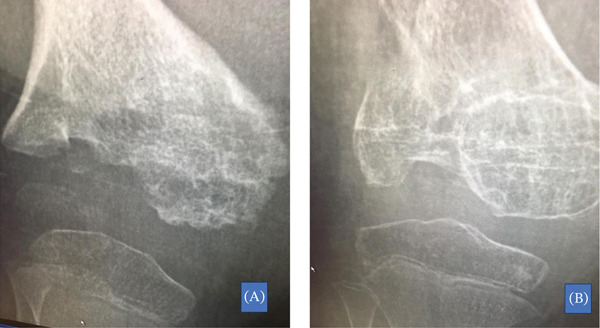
Twelve‐month follow‐up radiograph showing development of the lateral condyle and centralization of the (B) patella compared to the (A) preoperative x‐ray.
